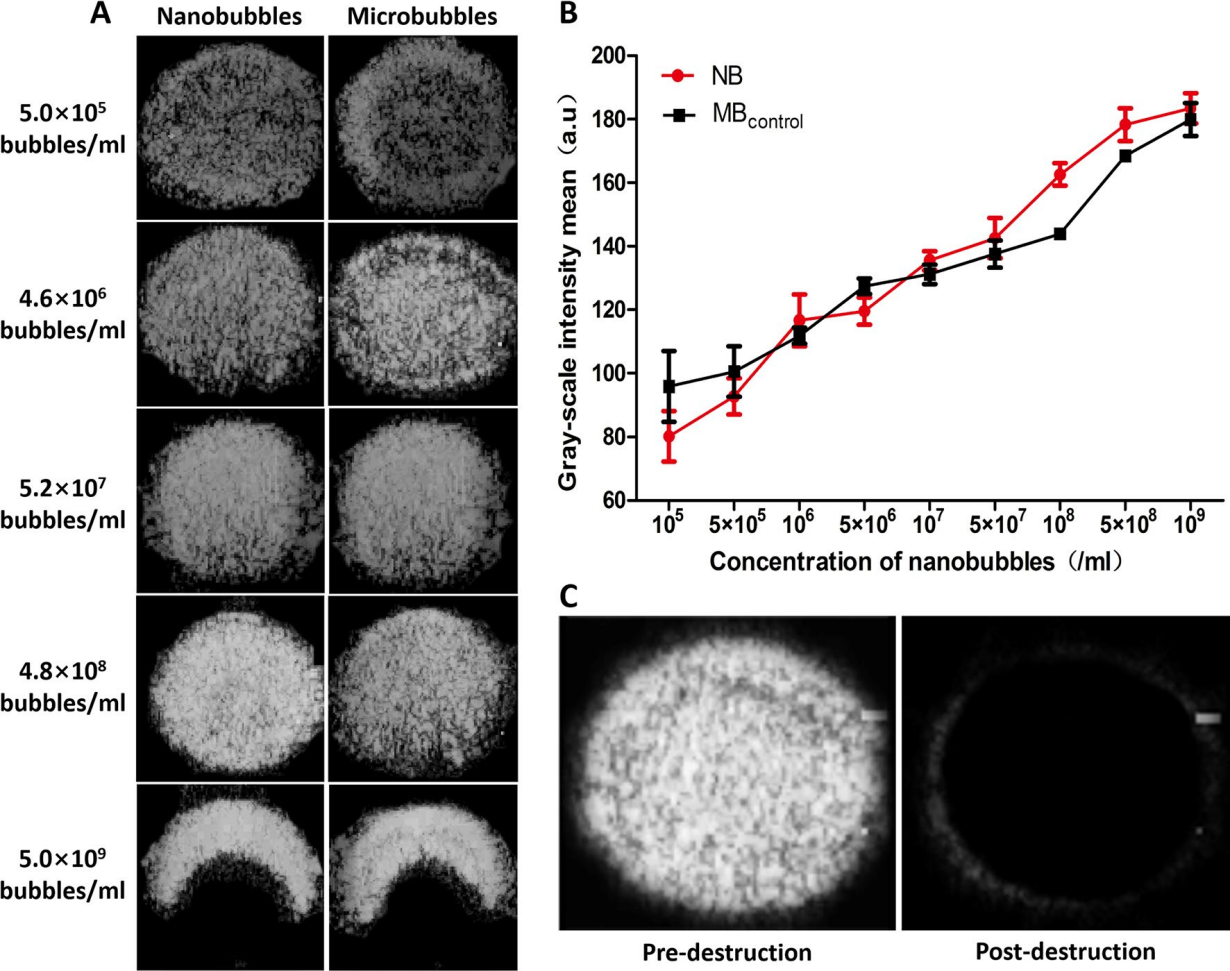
In vitro* ultrasound image enhancement. (A) Representative ultrasound images of nanobubbles and microbubbles *in vitro*. (B) Comparison of contrast intensity between nanobubbles and microbubbles at different concentrations. (C) Pre- and postdestruction of NBs at low-frequency ultrasound exposure.
